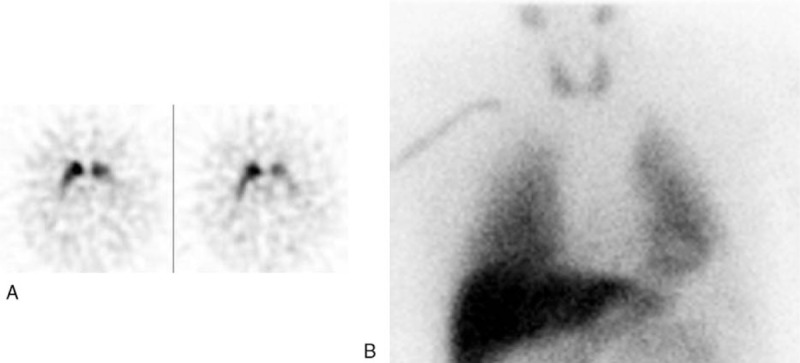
A 70-year-old male patient with uncertain parkinsonism, vascular lesions in basal ganglia at MRI, and partial response to dopamine agonist treatment. ^123^I-Ioflupane SPECT was pathological in left putamen nucleus (A) with also reduced BP value (1.9); ^123^I-MIBG cardiac scintigraphy was normal (B) with H/M values above cut-off both in early (1.8) and delayed (1.8) phases. The patient was finally classified as VP. H/M = heart to mediastinum ratio, MIBG = metaiodobenzylguanidine, SPECT = single photon emission computed tomography, VP = vascular parkinsonism.
